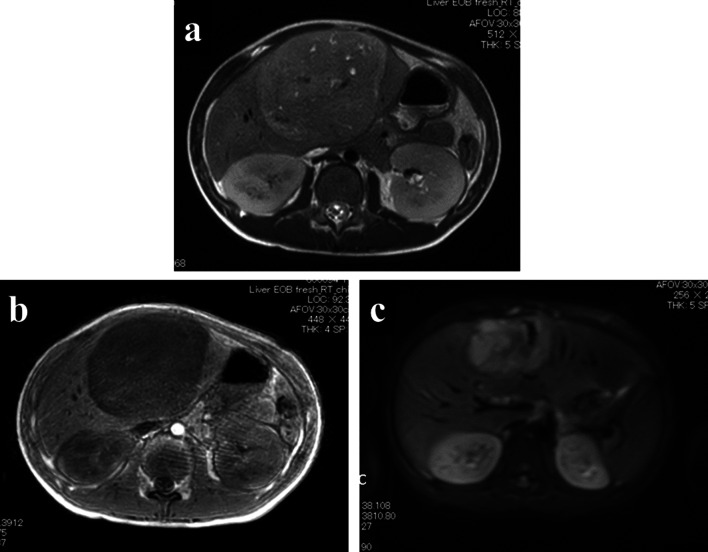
MRI findings. The tumor shows low intensity on T1-weighted imaging (a) and high intensity on T2-weighted imaging (b) with partial diffusion restriction (c)
